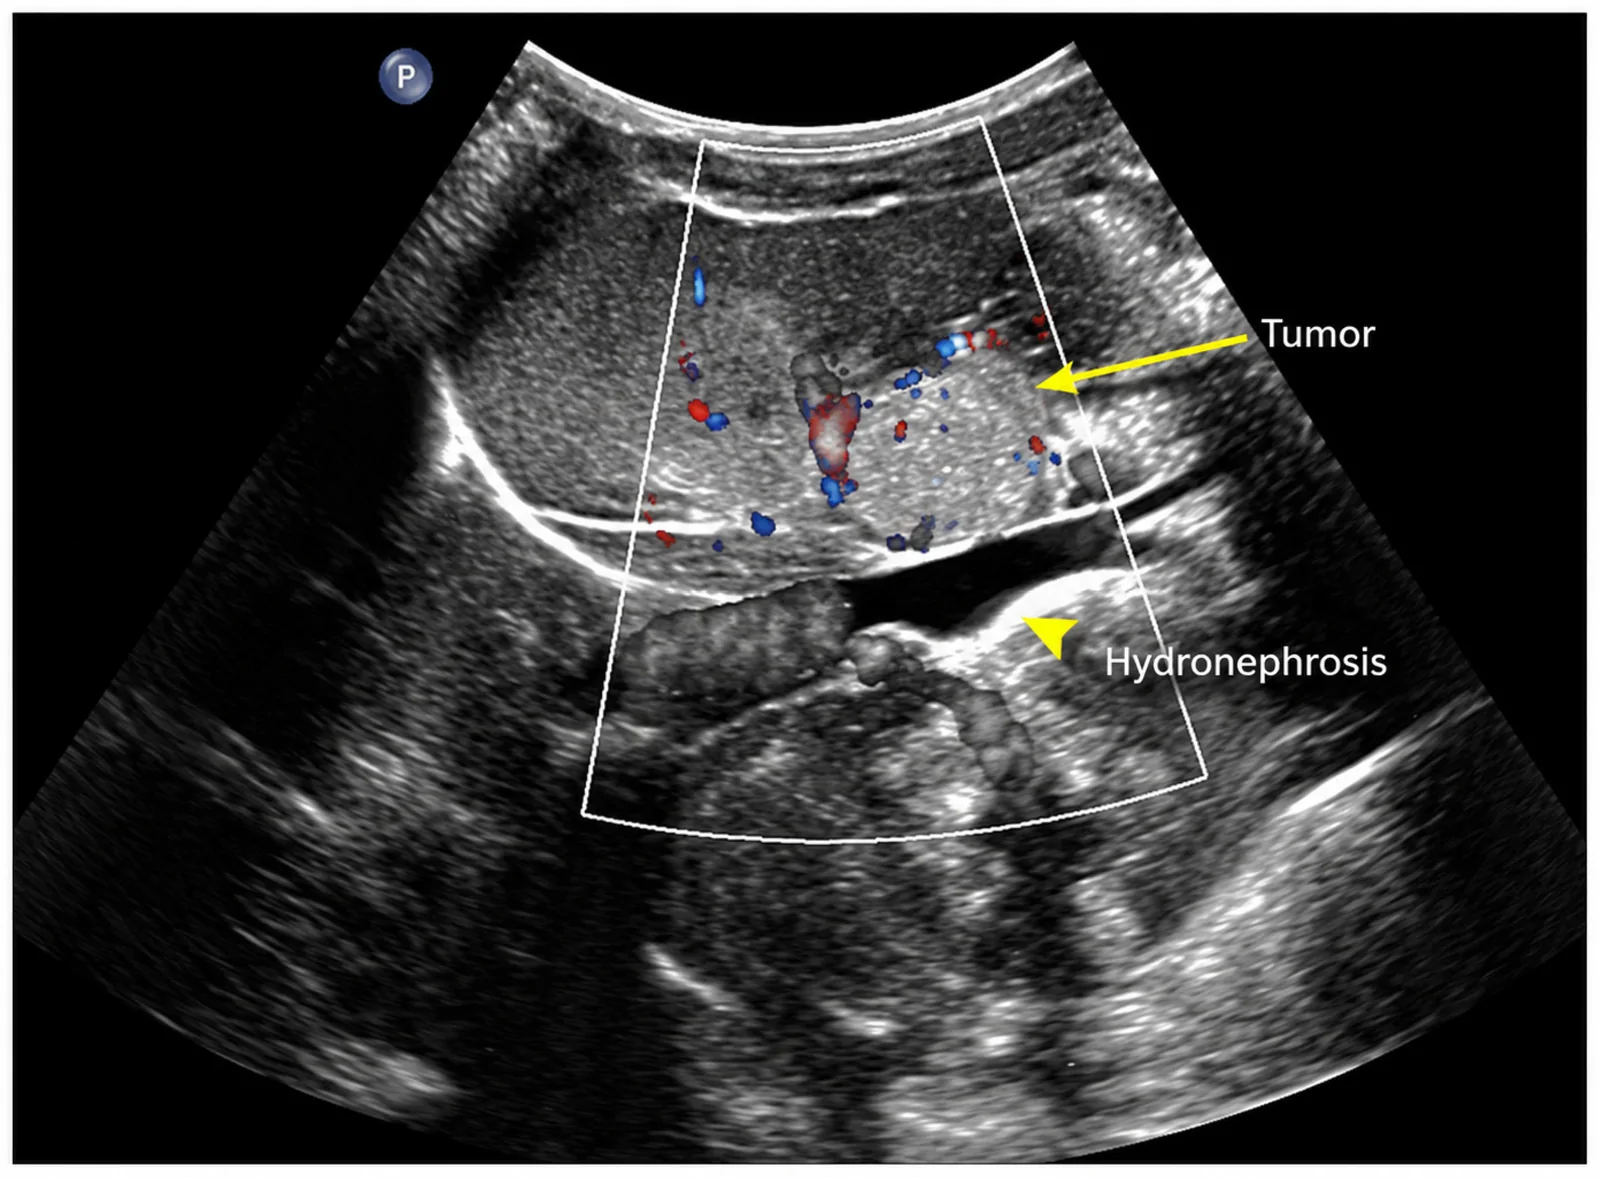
Postnatal abdominal ultrasonography demonstrating a right-sided suprarenal mass (approximately 17.7 × 14.9 mm) associated with ipsilateral hydronephrosis. The lesion is indicated by the arrow.
